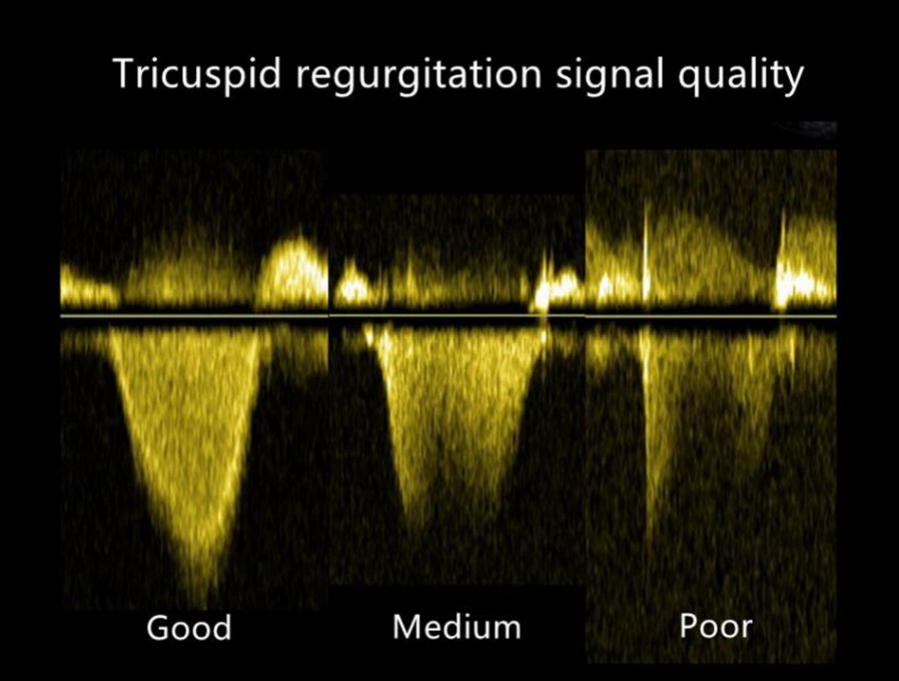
Classification of the TR signal quality using continuous-wave Doppler. Good signal quality, complete envelope; Medium signal quality, partial envelope; Poor signal quality, unreliable envelope or no signal
